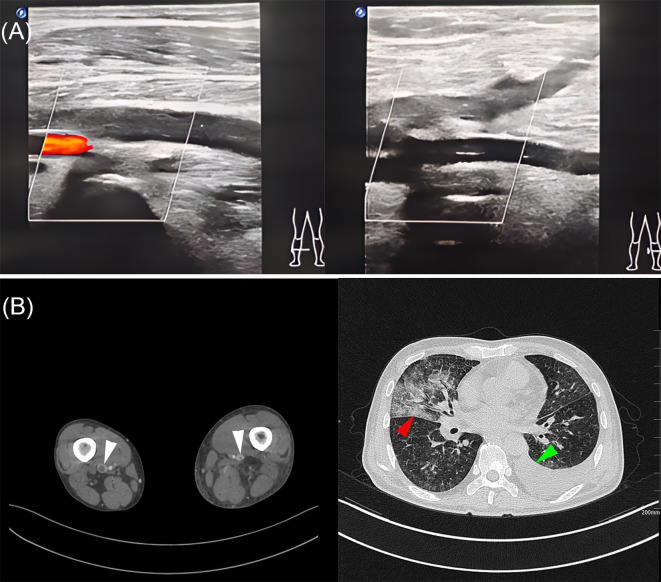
Imageological examination for this patient. (A) DVT in both lower extremities by color doppler ultrasound. (B) Imaged by CT, white arrowhead indicates thrombus, red arrowhead indicates pulmonary inflammation and green arrowhead indicates hydrothorax.
